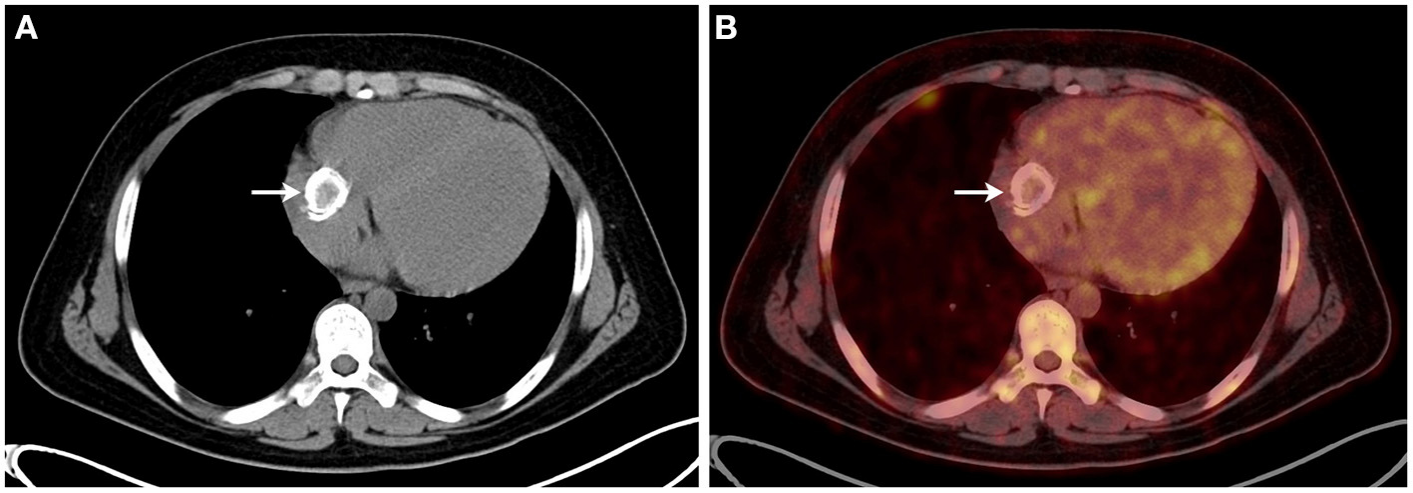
(A,B) The 18F-fluorodeoxyglucose positron emission tomography combined with computed tomography showed that a dense circular shadow was in the right atrium abutting the tricuspid valve (32 × 34 mm), and there was no increase in glucose metabolism.
